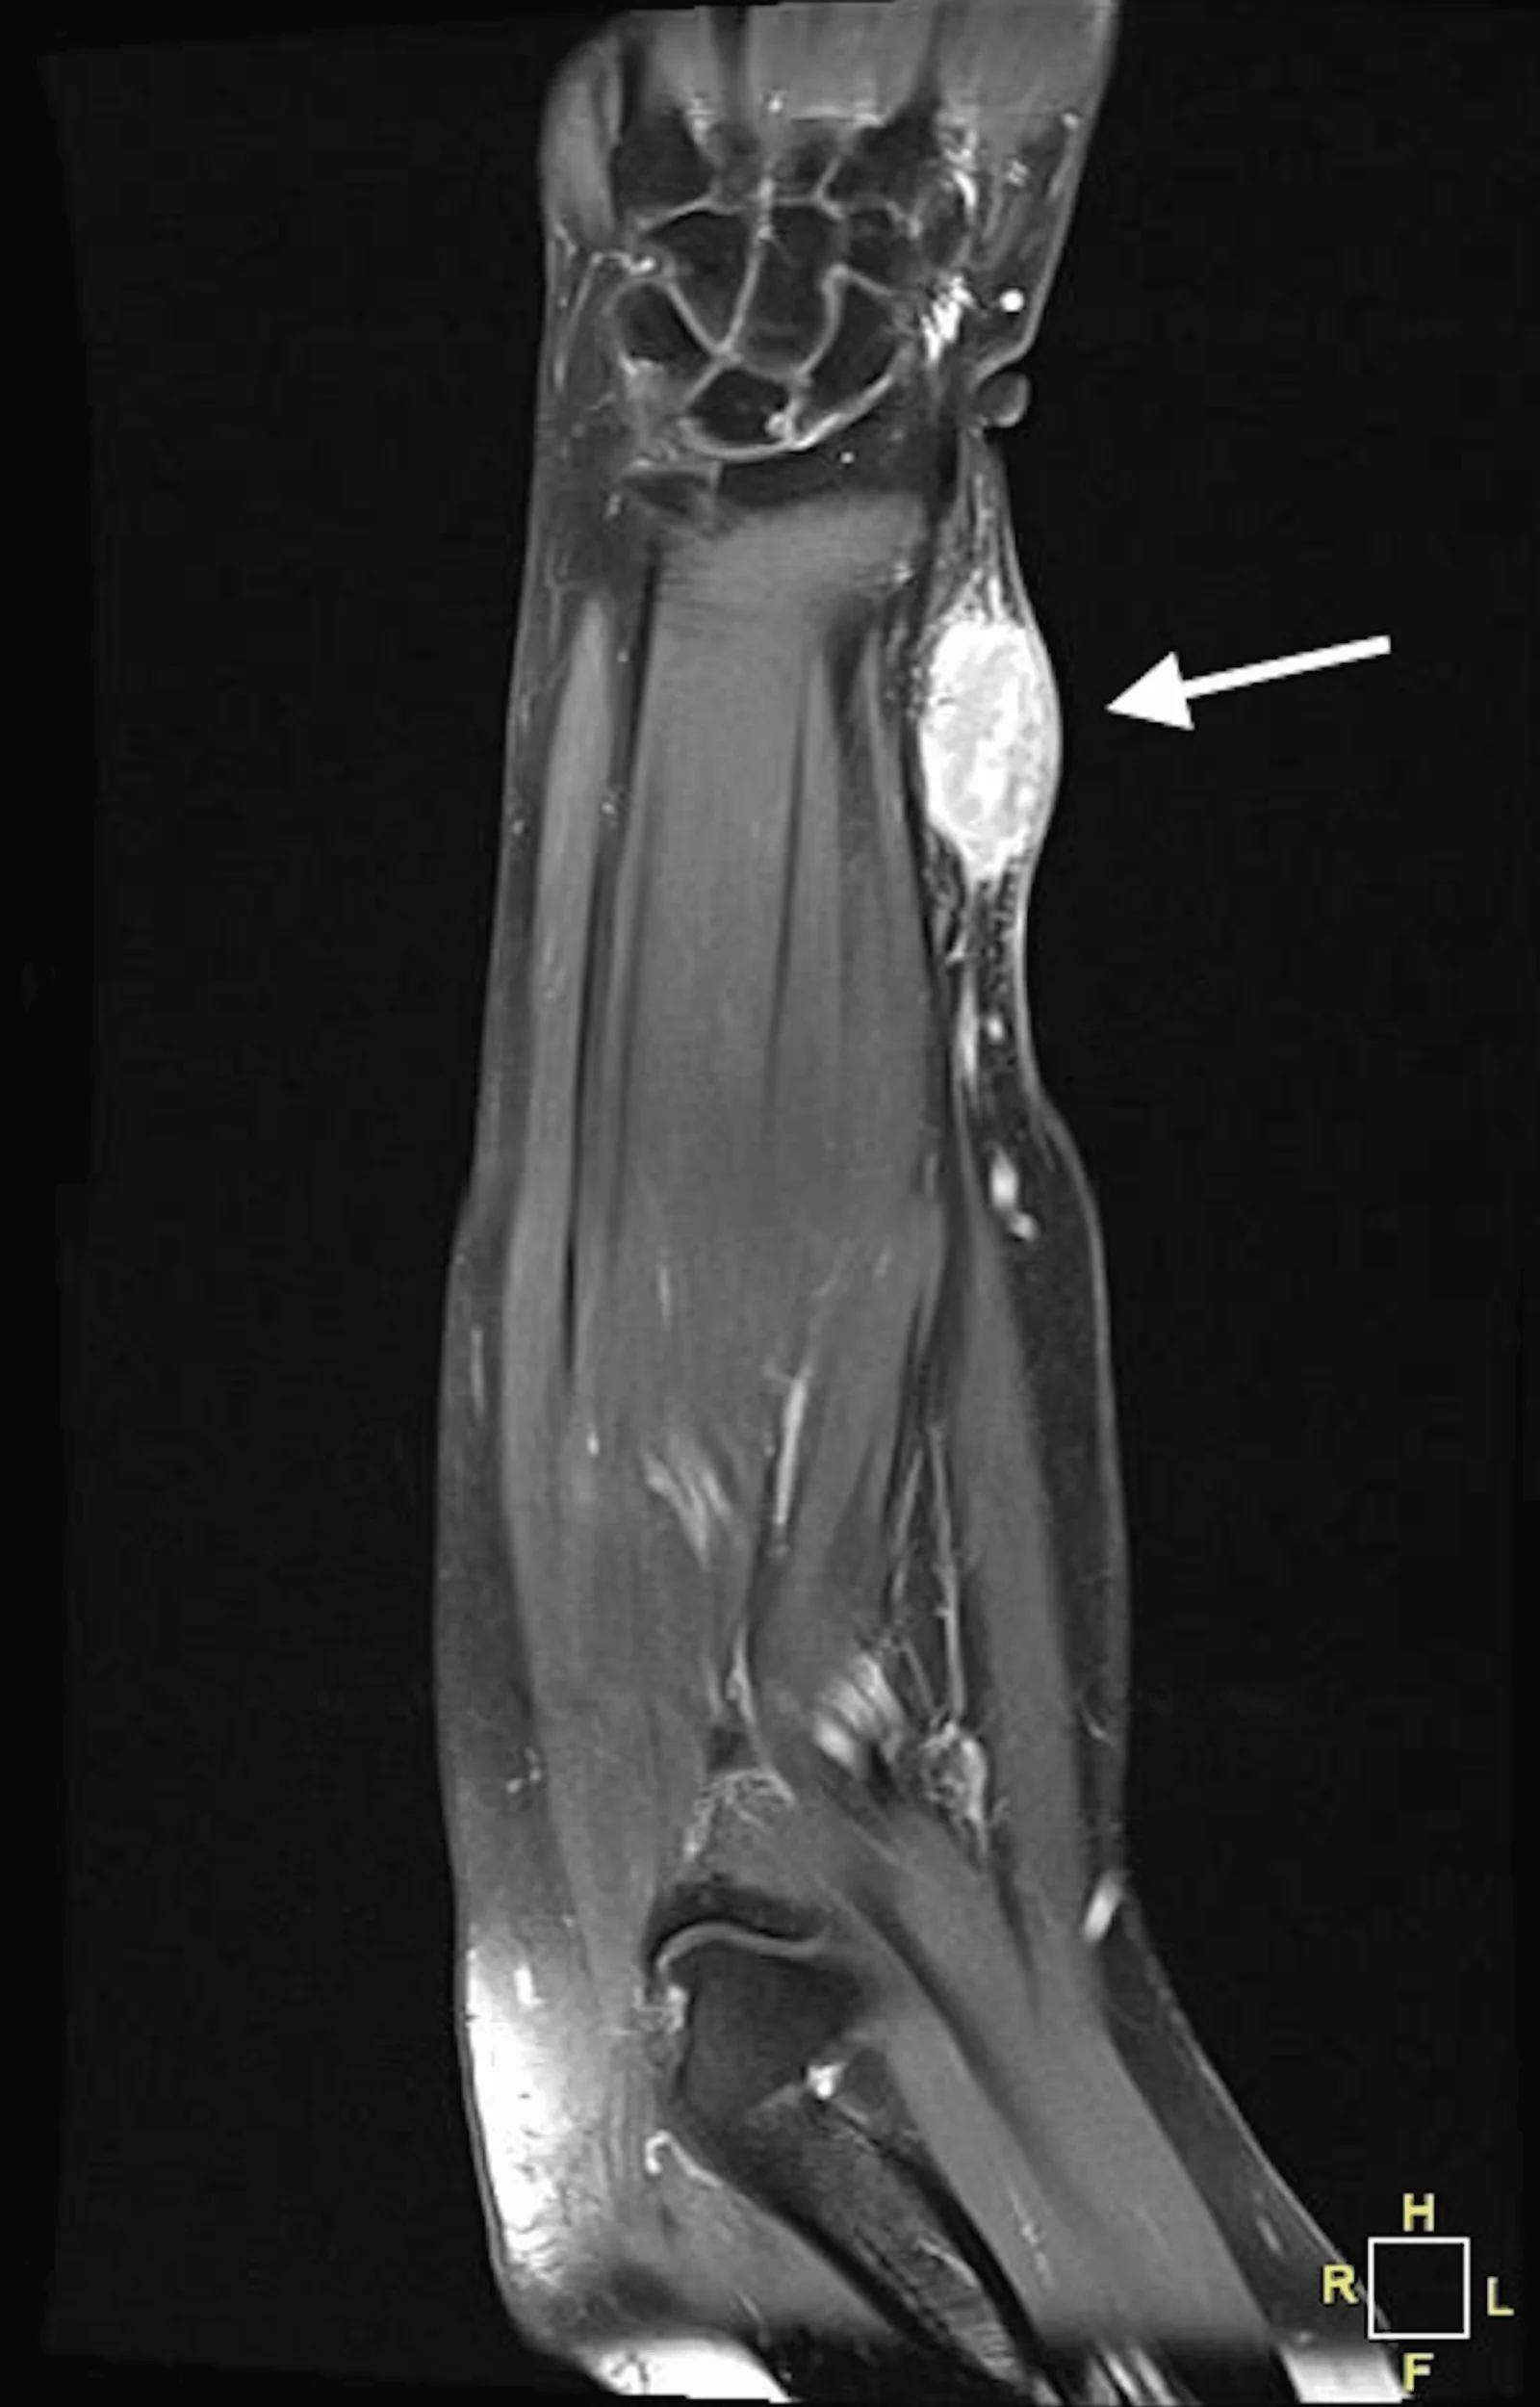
T1 coronal magnetic resonance image post IV gadolinium contrast of the right forearm showing a heterogeneous, lobular soft tissue mass in the subcutaneous fat, measuring 4.2 x 2.0 x 4.0 cm, with post-contrast enhancement most consistent with sarcoma. The lesion was confined to the subcutaneous tissues without involvement of the underlying muscle, tendon, or bone.
